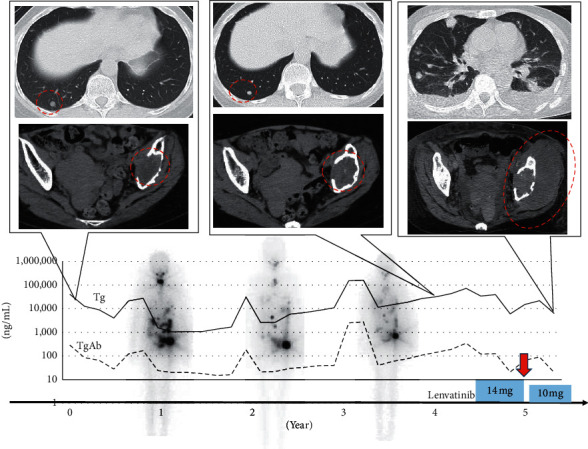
Clinical course of Case 1. The upper image is a chest computed tomography (CT) image. Although lung metastasis did not change after three courses of radioactive iodine (RAI) treatment, the patient's condition became progressive disease after anaplastic transformation and bilateral pleural effusion accumulated. The lower image is a CT image of the pelvis, and the left iliac metastasis increased rapidly after anaplastic transformation. The graph below the image shows the transition of thyroglobulin and antibodies, the timing of lenvatinib treatment and the dose. Whole body scintigraphy images after three RAI treatments are also shown in the background. RAI uptake is decaying. The red dotted circle indicates the lesion. The red arrow indicates the time of anaplastic transformation.
